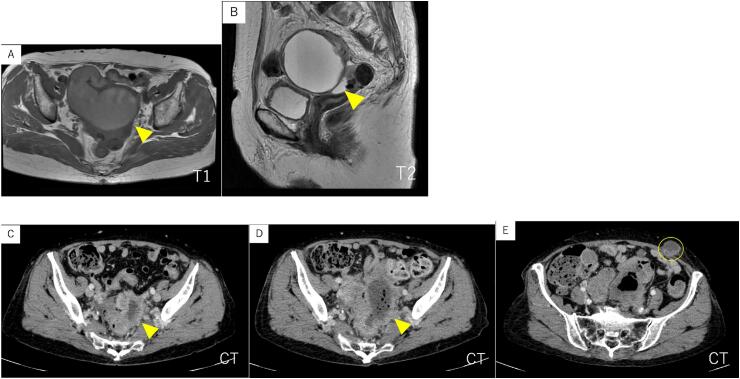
Images of the patient. A: MRI, T1-weighted image. Sausage-shaped fallopian tube present. B: MRI, T2-weighted image. Cystic lesion present ventral and cranial to the uterus. C: CT, fluid accumulation present on the left side of the sigmoid colon. D: CT, increased fluid accumulation on the left side of the sigmoid colon, with gas present internally. E: CT, mass formation presence at the left lower abdominal port site.
